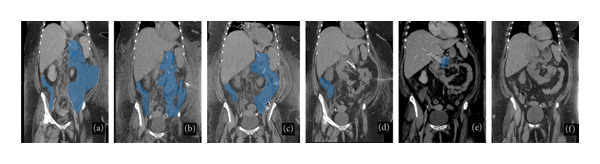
Progression of mixed density collections in the perigastric, left pericolic, and right pericolic region (shaded in blue) (a–d) throughout the treatment process. (a) Initial CT abdomen after 12‐Fr PCD. (b) 1 month after placement of 3 PCDs with significant ascites. (c, d) After H_2_O_2_ flush initiation with 1‐week and 1.5‐month follow‐up, respectively. (e) 3‐month follow‐up after treatment shows residual 3.7‐cm pancreatic cyst highlighted in blue. (f) S1‐year follow‐up with no residual disease.
